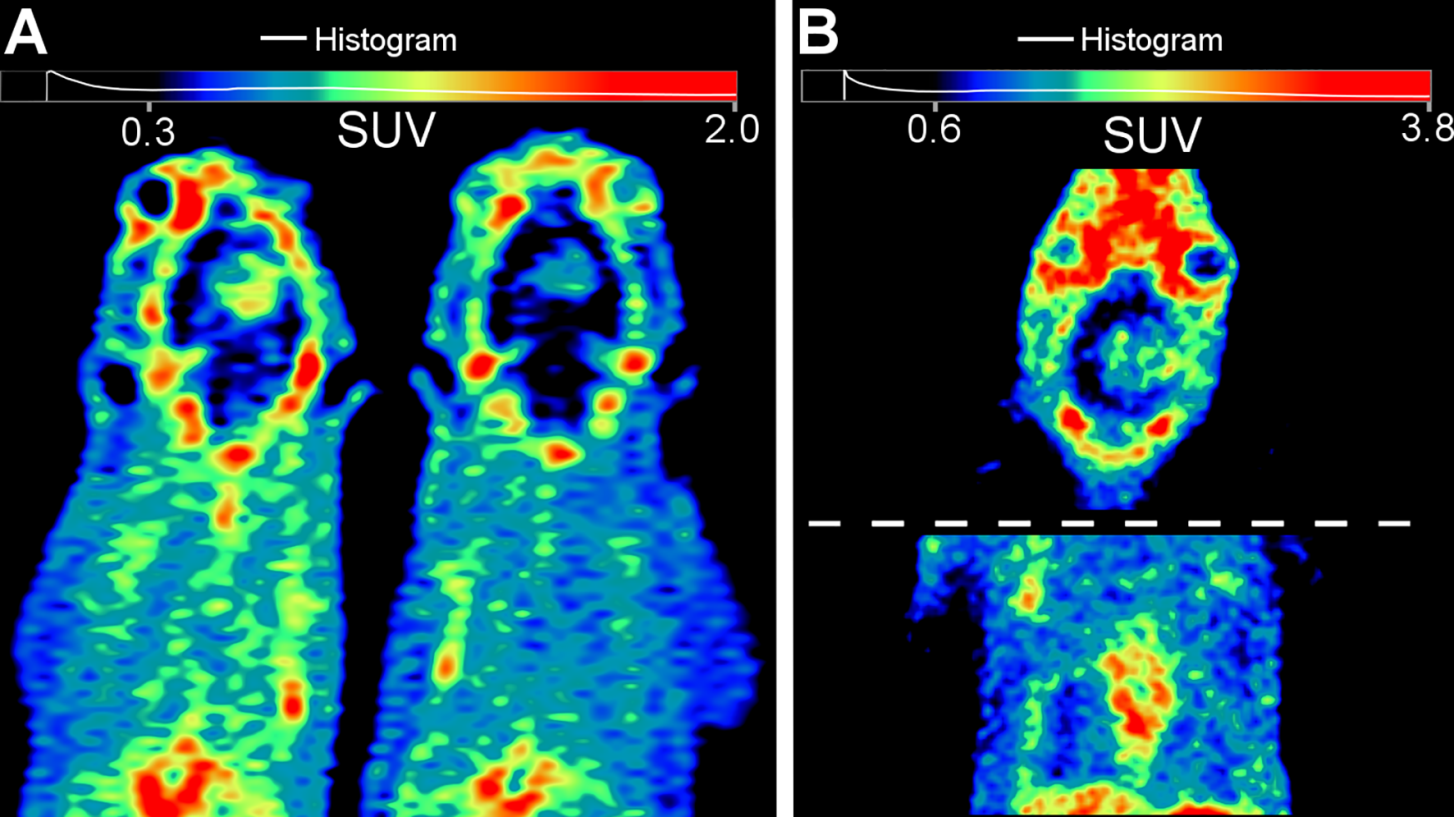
Sample visual camera-comparison of coronal [^18^F]FGln PET image slices with tri-cubic interpolation of time-weighted mean frames from 4 – 40 min post-injection. (A) Inveon PET/CT reconstructed with manufacturer recommendations (2 OSEM3D and 18 MAP iterations) with both subjects injected with approximately 15.2 MBq each. (B) Molecubes camera system (reconstructed with manufacturer recommendations (30 OSEM3D iterations) with the subject injected with 4.2 MBq. *note: To show similar body areas, the head and torso were split in (B) due to body positioning and gantry differences between each camera not showing the same region in a single coronal-plane slice. Additional organ and image comparisons presented in the Supplementary Materials Results section.
